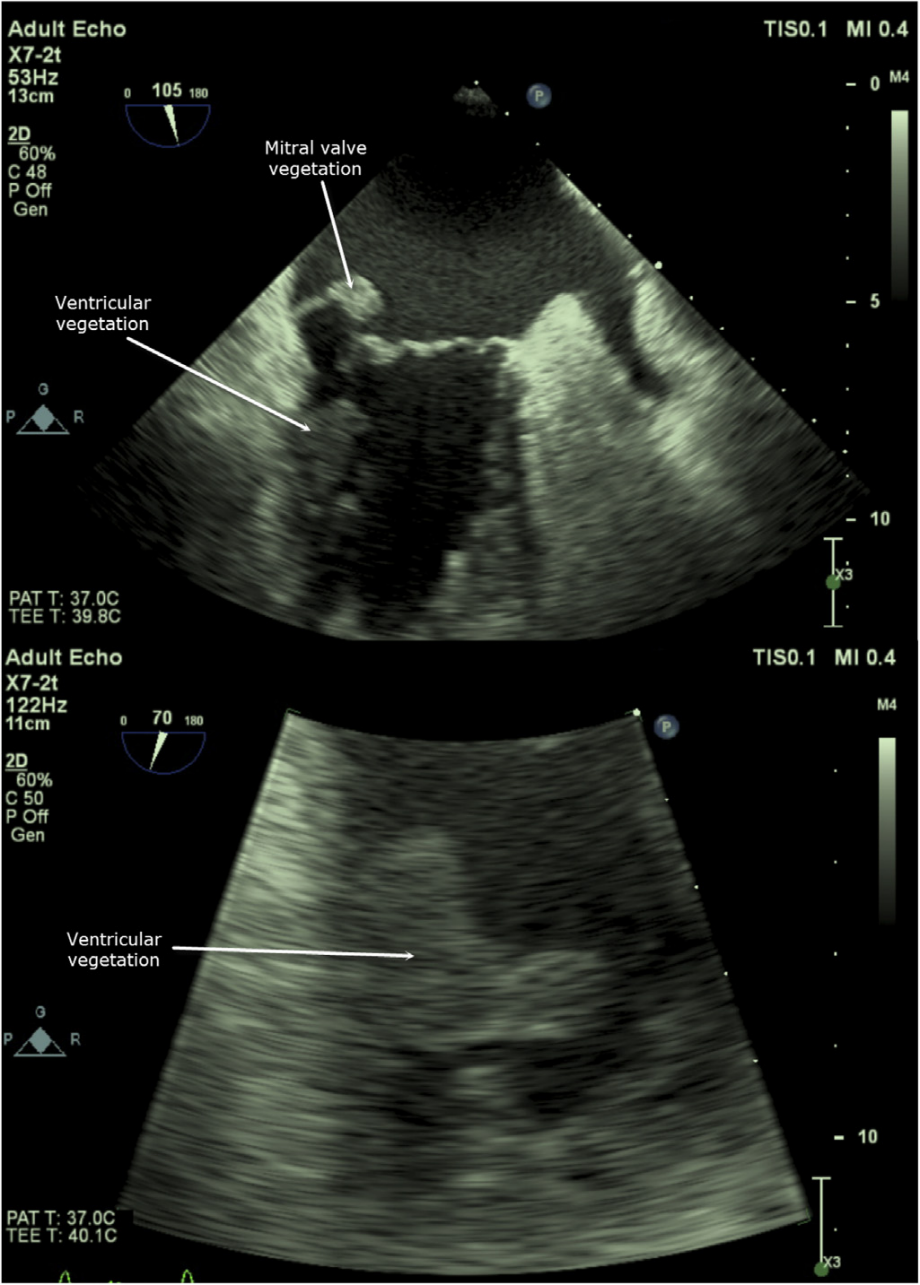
Transesophageal echocardiogram showing a pedunculated mitral valve vegetation and multi-lobed mid-ventricular wall vegetation.
